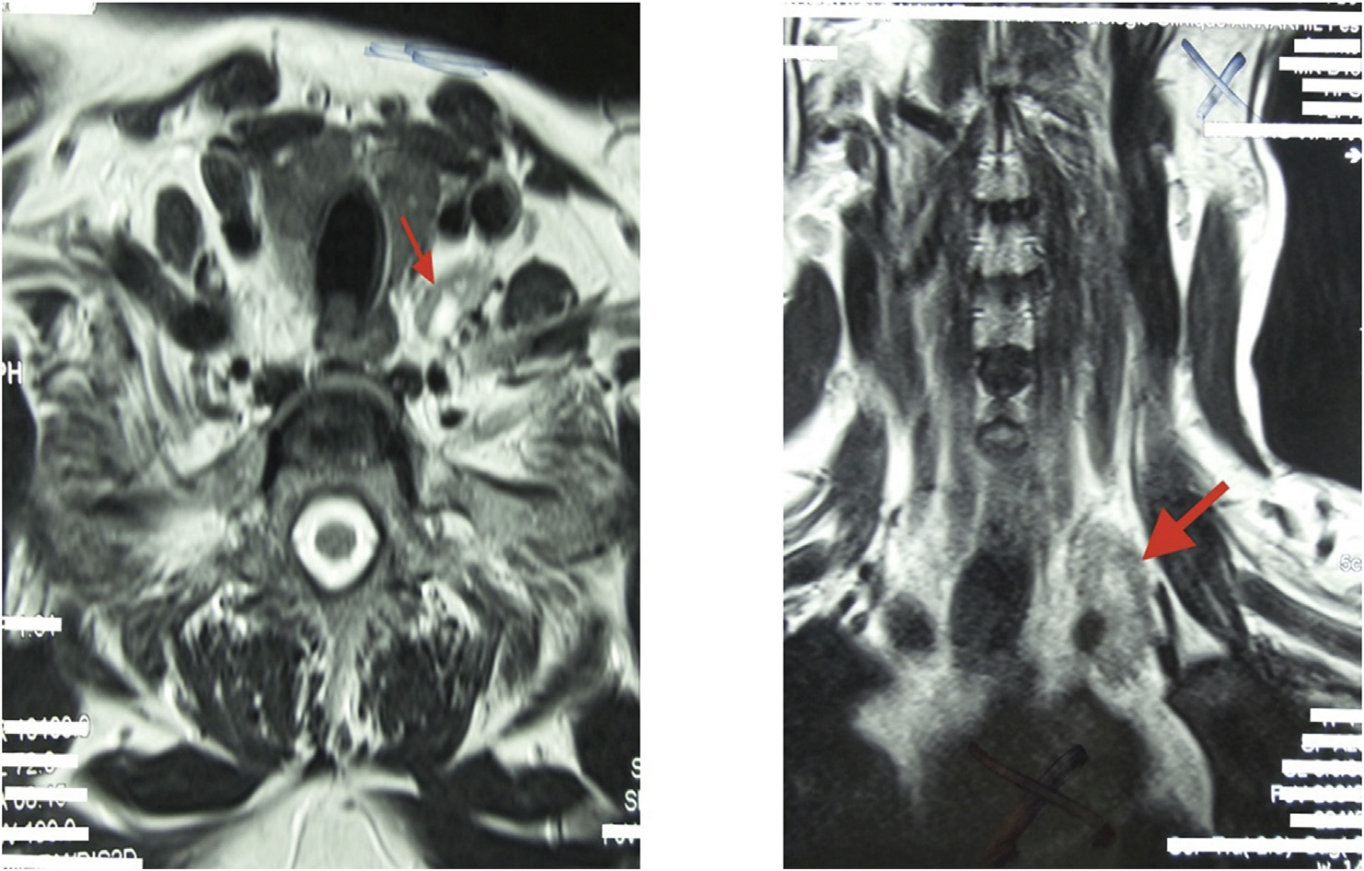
CT-scan showing interjugulo-carotidian mass corresponding to a parathyroid adenoma.
